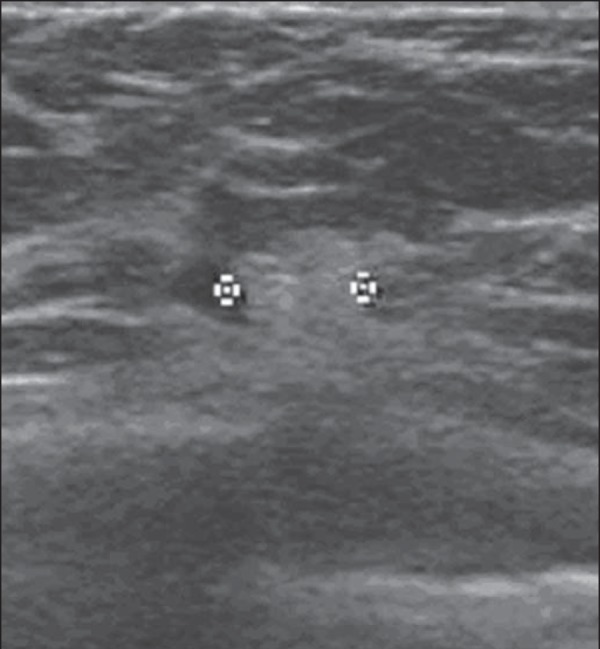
Invasive ductal carcinoma*. Ultrasonography showing
